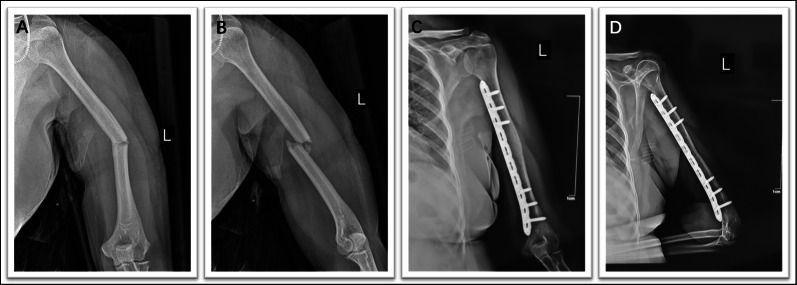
A typical case. The patient fell while walking down the stairs and sustained a middle fracture of the humerus (left side). A–B Preoperative X-rays. C–D X-rays taken 4 months after surgery
